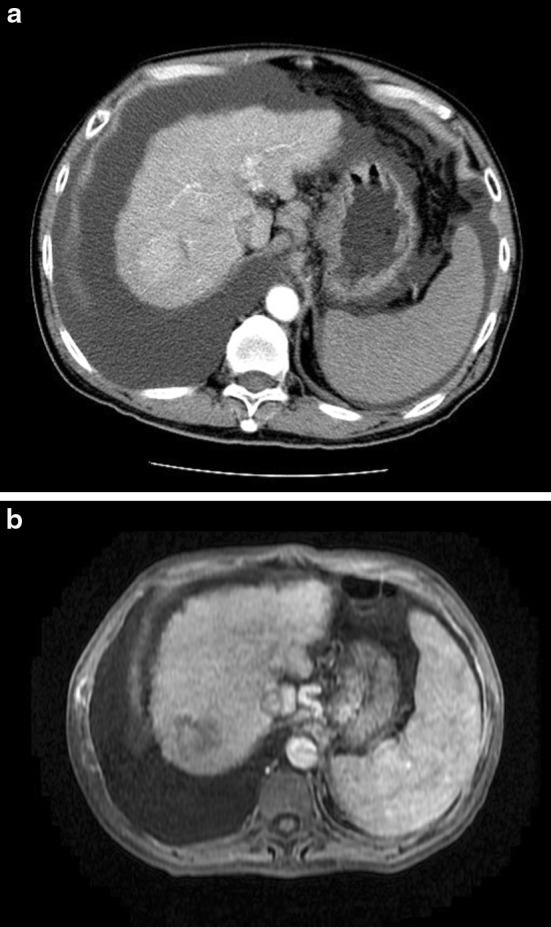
a Computed tomography scan showing a hepatocellular carcinoma at the dome of liver. The liver is of Child-Pugh C cirrhosis with gross ascites. b Magnetic resonance imaging scan of the same patient 1 month after high-intensity focused ultrasound (HIFU) ablation. Complete ablation is noted in this scan. The patient’s liver functions did not deteriorate from their pre-HIFU status
